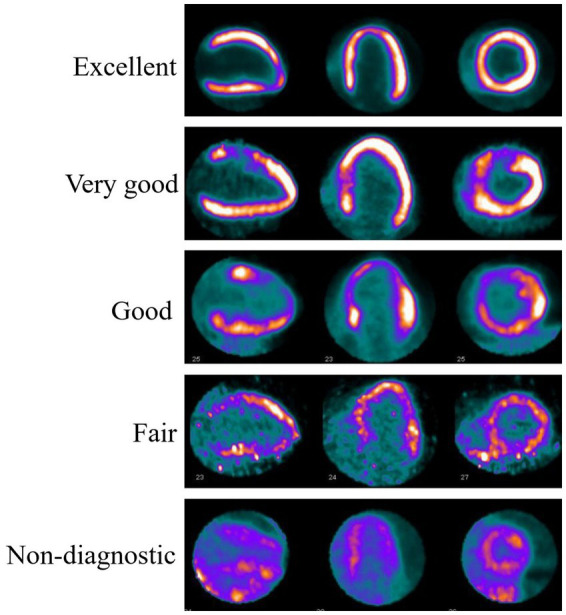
Myocardial ^18^F-FDG image quality was evaluated according to 5-point score system.
